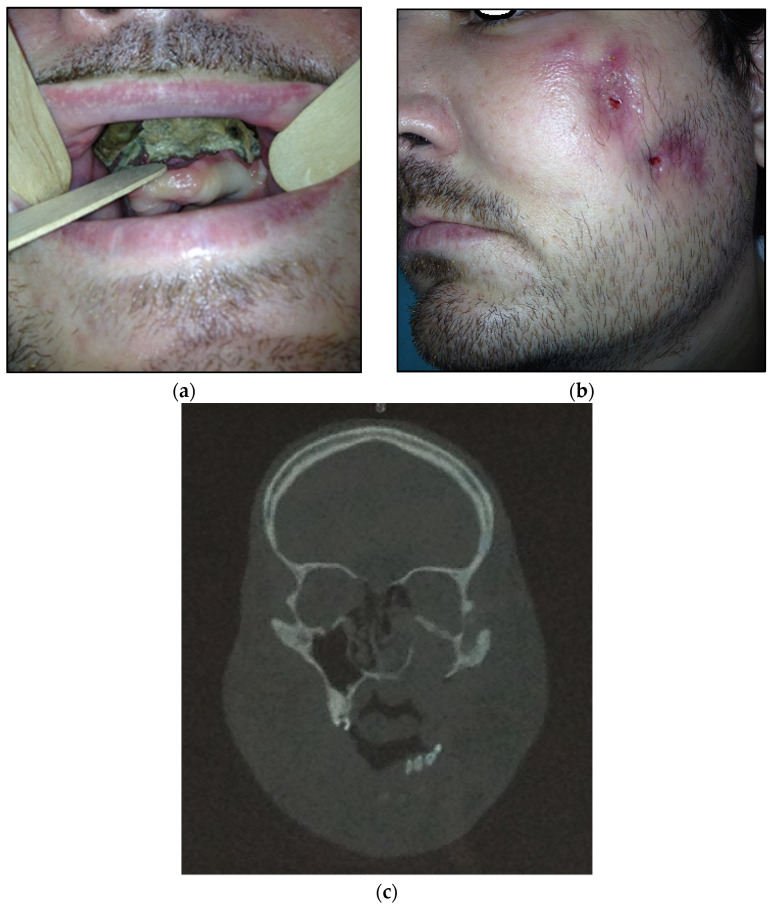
(a) Patient with type 3 toxic phosphorus osteonecrosis. Intraoral view; (b) extraoral view, (c) multi CT-scan of patient’s skull before operation.
